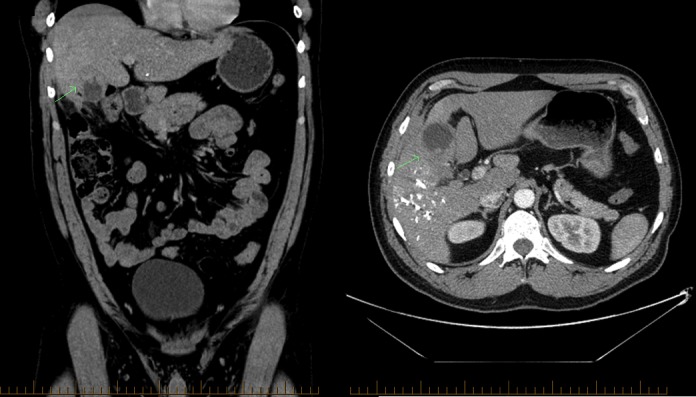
Initial staging CT of the abdomen and pelvis with contrast Coronal (left) and transverse (right) view showing extensive gallbladder mass prior to starting chemotherapy.
